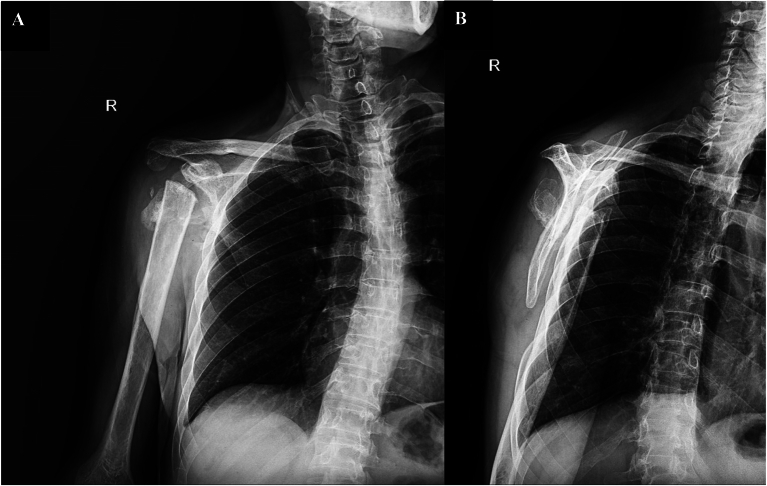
Radiographic image (anteroposterior (A) and scapular Y-view (B)) of the right shoulder showing extensive bone resorption in the right humeral head and joint destruction consistent with Charcot shoulder.
